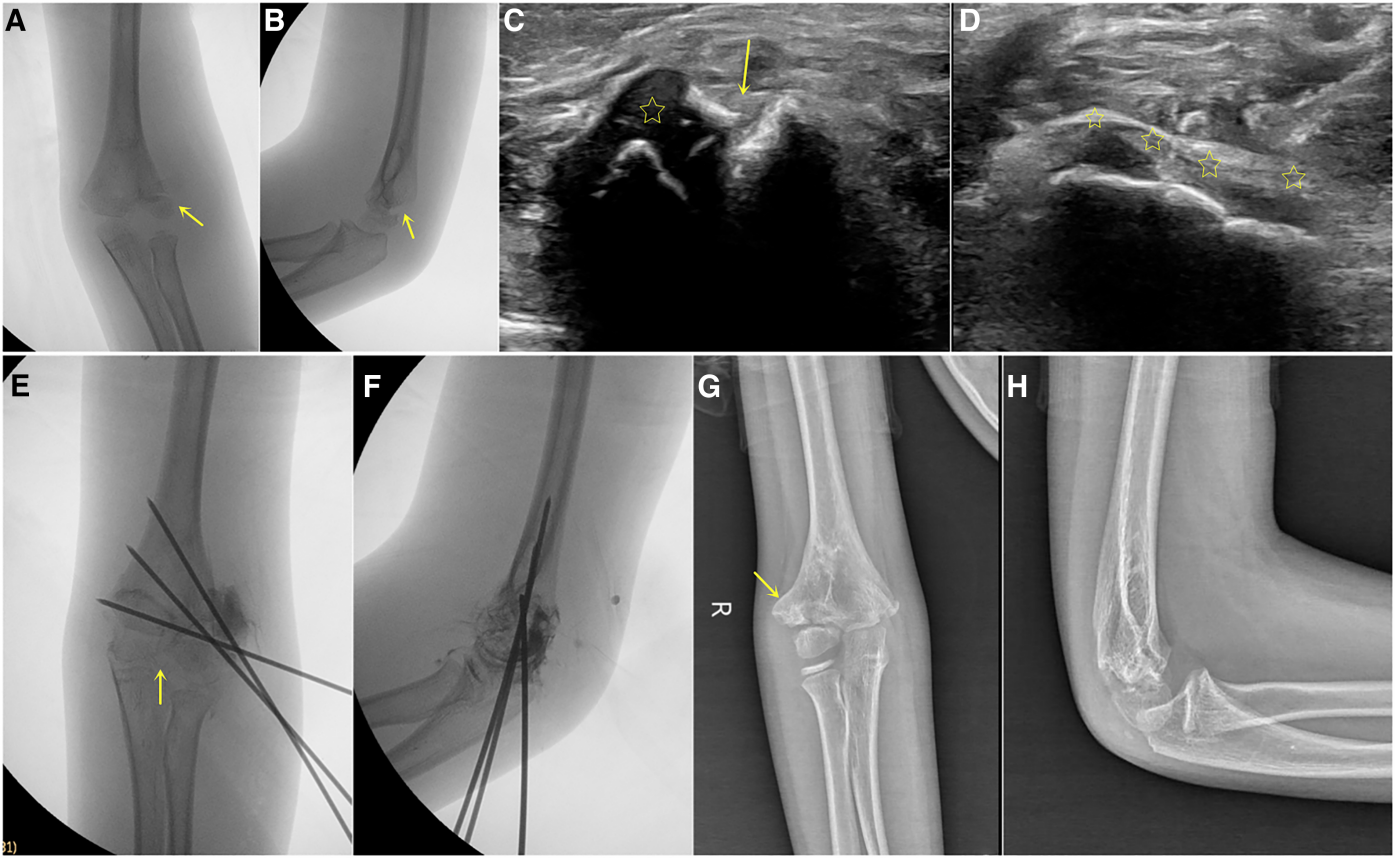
Boy, 8 years old, with right humeral lateral condyle fracture, song type 4. (A,B), preoperative x-ray showed lateral displacement of the fracture, with obvious displacement but no rotated (arrow). (C) Preoperative ultrasound showed displacement of fragment (arrow indicates fracture line, asterisk indicates fracture fragment). (D) After reduction, continuous cartilage hinges were observed (arrow). (E,F) Postoperative imaging showed continuous cartilage hinge (arrow) and divergent Kirschner wires fixation. (G,H) After 20 months of postoperative follow-up, obvious lateral spur (arrow) was observed, and the elbow function was good.
